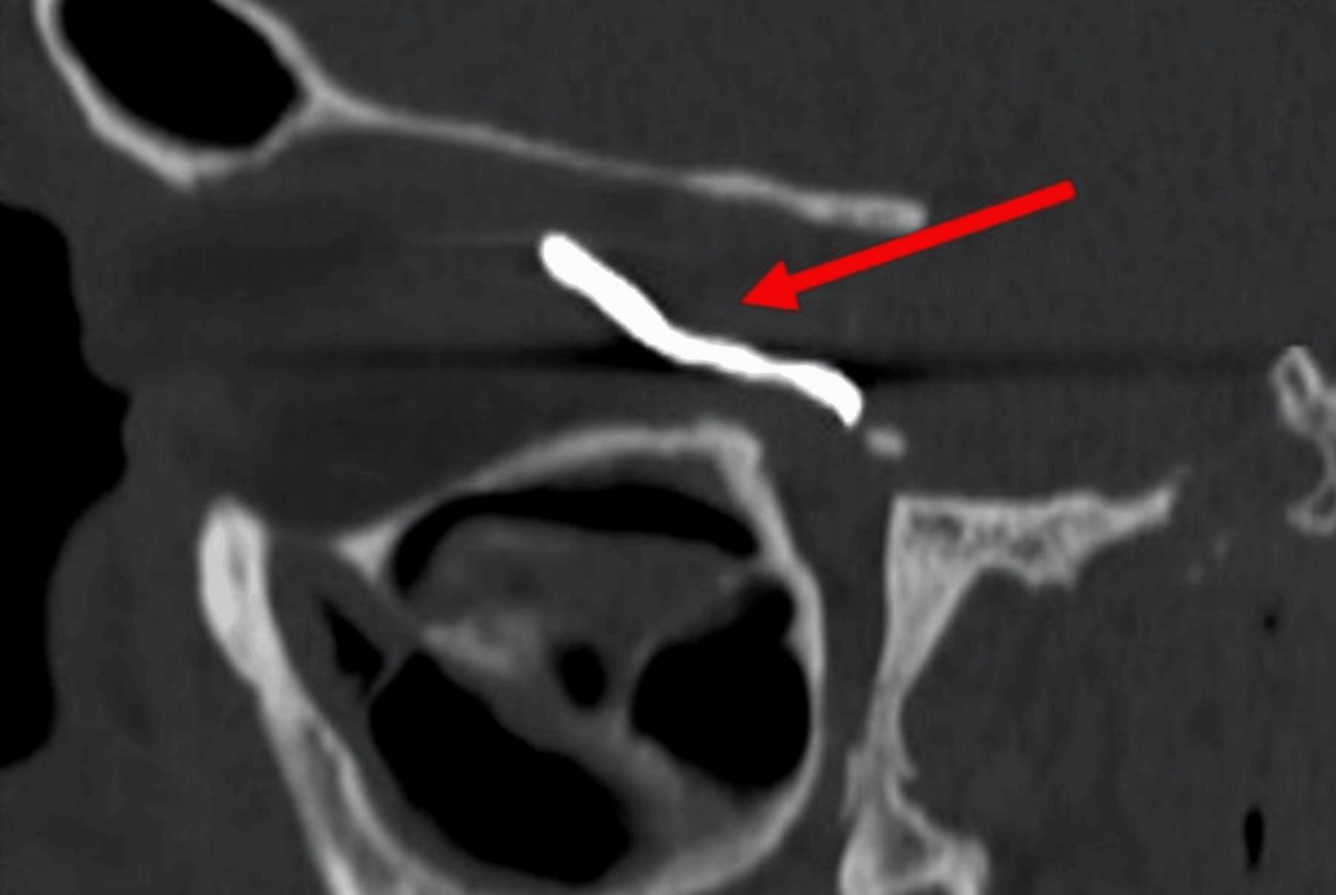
Preoperative computed tomography (CT) scan of the patient's right orbit The red arrow indicates a metallic foreign body located within the medial orbit. The foreign body appears as a hyperdense structure, consistent with the patient's clinical presentation of persistent pain and diplopia. This image emphasizes the importance of thorough imaging in cases of suspected retained intraorbital foreign bodies.
